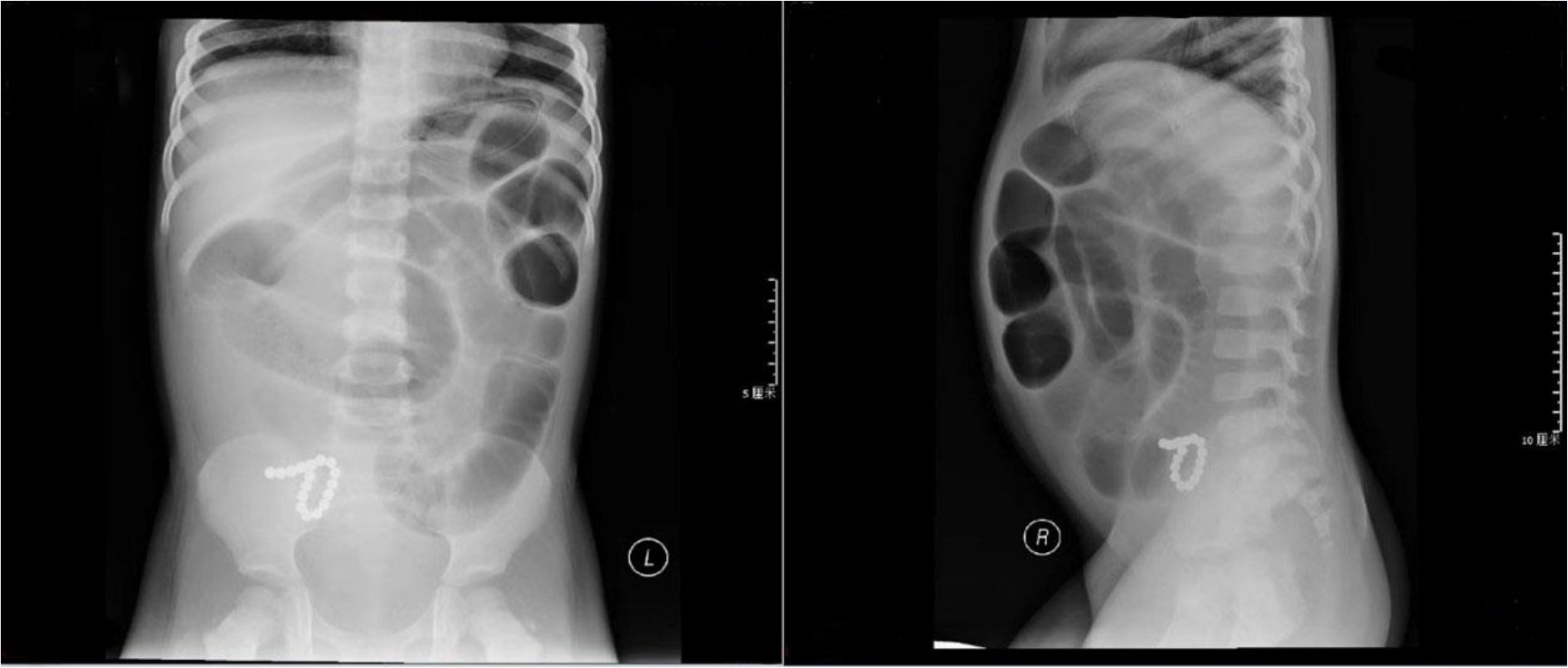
Anterior and lateral X-ray views of a patient who ingested a string of magnetic beads.
